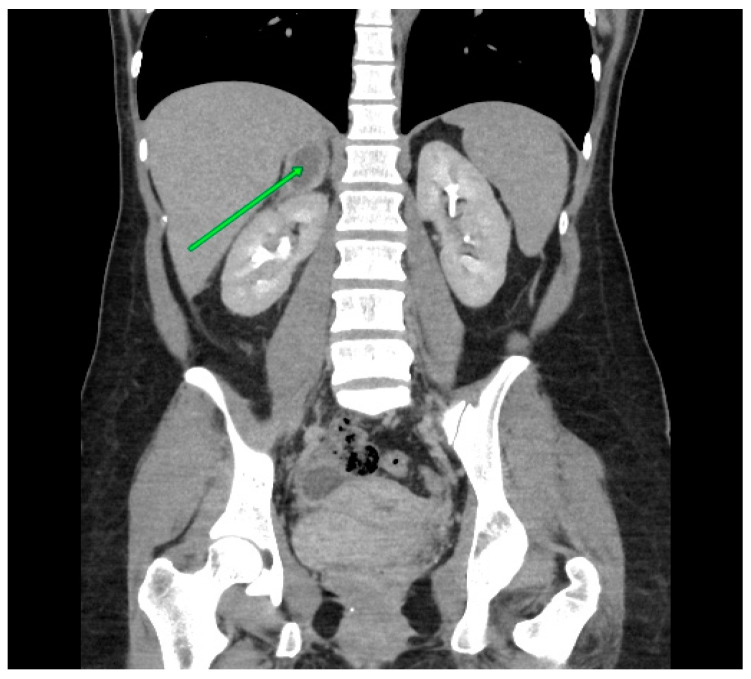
Abdominal CT scan. A hematoma is present in the projection of the right adrenal and is markedly smaller in size than that observed in the MRI performed three months ago.
